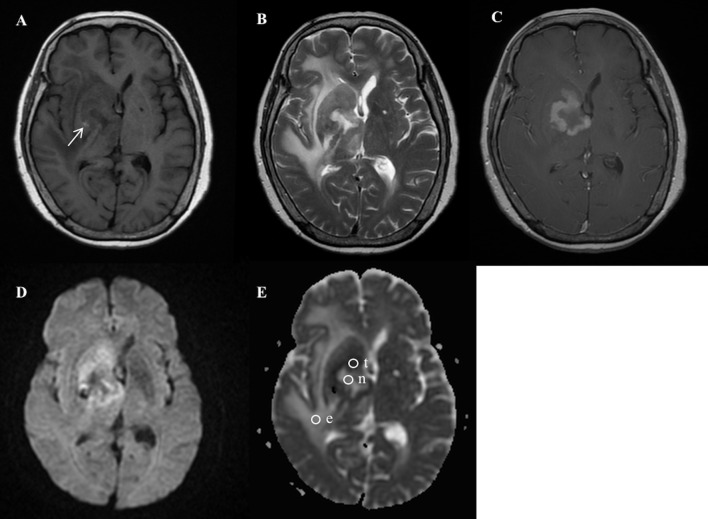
A 74-year-old female with pathologically-proven primary cerebral lymphoma after biopsy. (A) Axial T1-weighted MR imaging (T1WI) and (B) T2-weighted MR imaging (T2WI) showed a heterogeneous tumor mass with central tumor necrosis and peritumoral edema involving the right basal ganglia, right internal capsule, and right thalamus. Spot hemorrhage in the tumor mass is shown as hyperintensity (arrow) on T1WI (A). (C) Axial contrast-enhanced T1WI revealed rim-enhancing mass in the right basal ganglia and internal capsule. (D) Diffusion-weighted imaging (DWI) showed a ring-shaped hyperintense tumor mass, central hypointense necrosis, and isointense peritumoral edema. (E) Apparent diffusion coefficient (ADC) map showed ROIs (circles) in the tumor necrosis (n), the most strongly-enhanced tumor area (t), and the peritumoral edema (e). Measured ADC (b = 1000 sec/mm^2^) in the tumor necrosis (ADCn) is 2.44 x 10^−3^ mm^2^/s; the most strongly-enhanced tumor area (ADCt), 0.84 x 10^−3^ mm^2^/s; the peritumoral edema (ADCe), 2.52 x 10^−3^ mm^2^/s.
